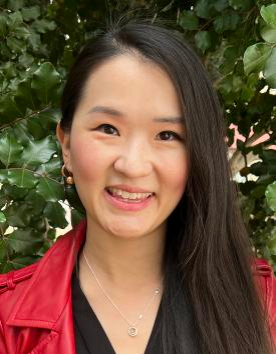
Jessica Yao is a Cardiology Fellow and PhD candidate with a special interest in Cardio-obstetrics and echocardiography.
